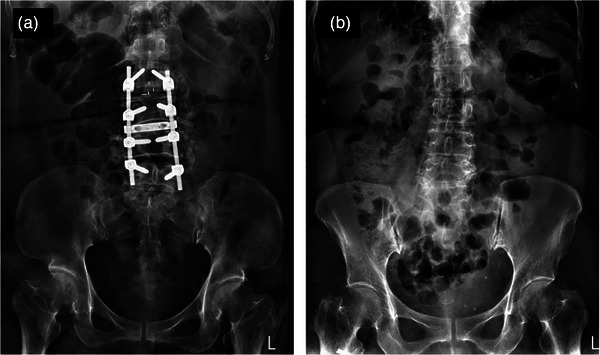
The KUB radiographs demonstrating the overexposure in a female patient with metal implant at the lumbar spine, (a) exposed at 84 kVp and 160 mAs, and normal exposure in another BMI‐matched female patient without metal implant, (b) exposed at 80 kVp and 70 mAs.
